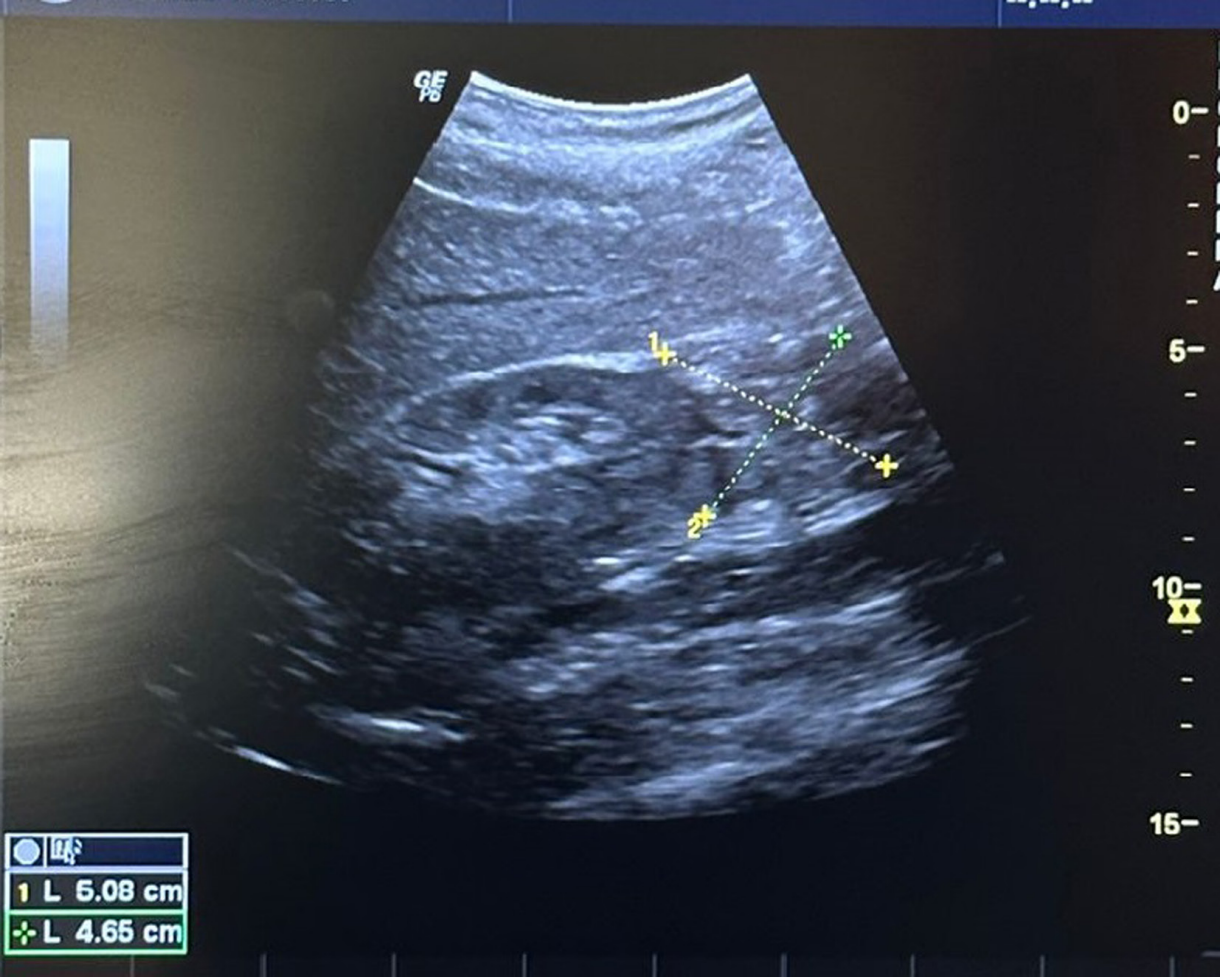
Ultrasound of the soft tissues showing a heterogeneous gluteal collection within the left gluteal muscle, measuring 5.08 cm × 4.65 cm.
